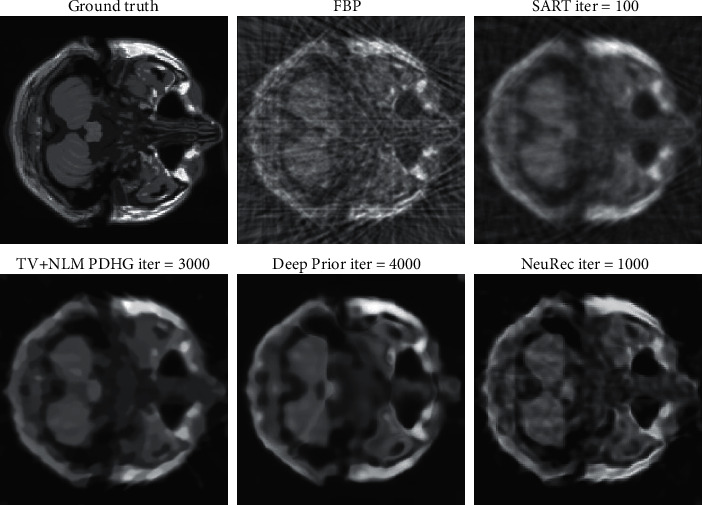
MRI reconstruction comparison.
